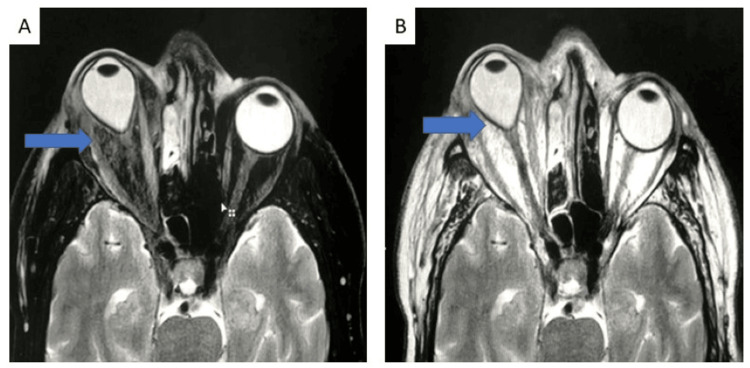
Orbital involvement: case 1 Axial MR image (T2FS/T2 sequences) shows thickening of lateral and medial rectus muscles with extensive fat stranding in the intra- and extraconal regions and muscle edema, resulting in distortion of the right globe (guitar pick sign) with proptosis.
